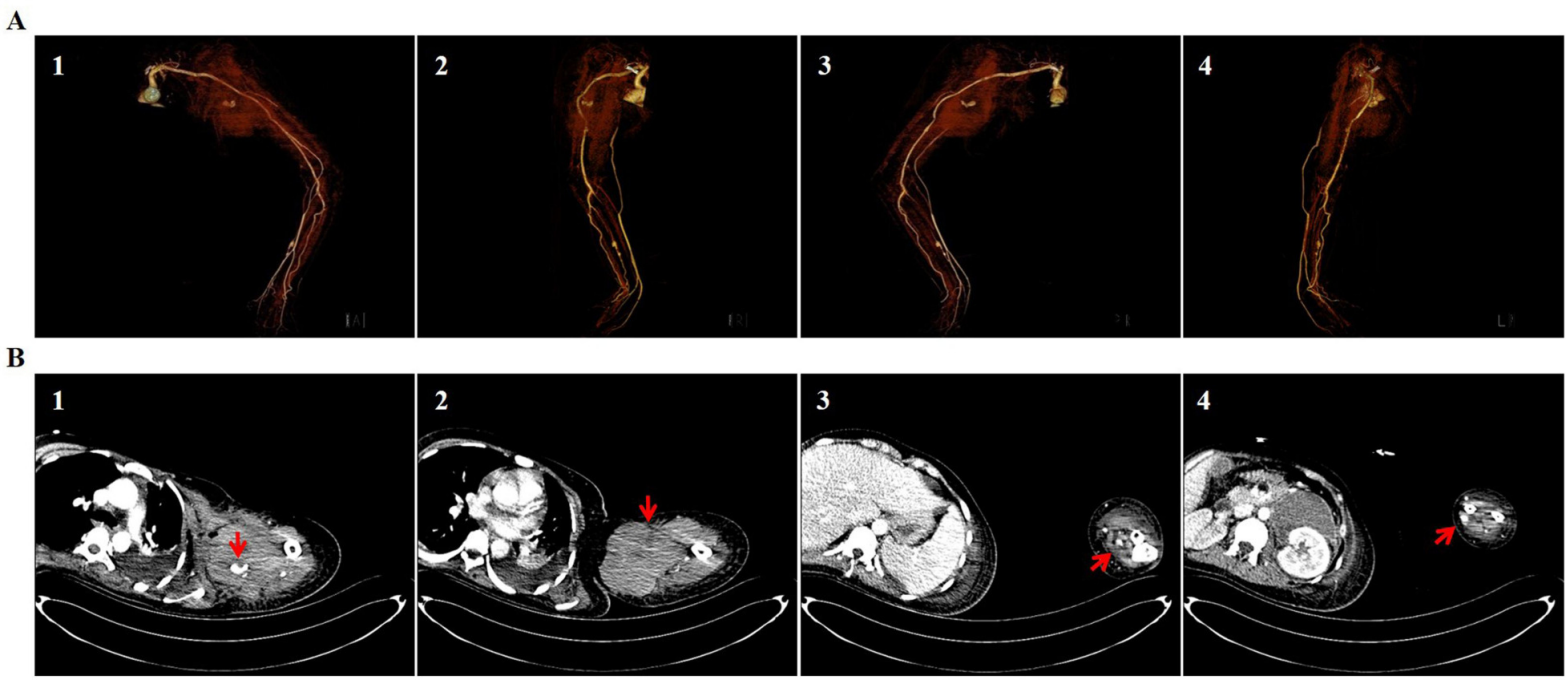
Fibromuscular dysplasia resulted in axillary artery pseudoaneurysm. A CT angiography found soft-tissue hematoma secondary to axillary artery pseudoaneurysm, small proximal brachial artery aneurysm, aneurysms, and stenoses in the proximal radical artery. The three-dimensional CT angiographic images are in the anterior (A1), right (A2), posterior (A3), and left (A4) views. The cross-sectional CT images indicate false aneurysm rupture (B1), hematoma (B2), brachial artery aneurysm (B3), and radical artery aneurysm (B4) (indicated by arrows).
